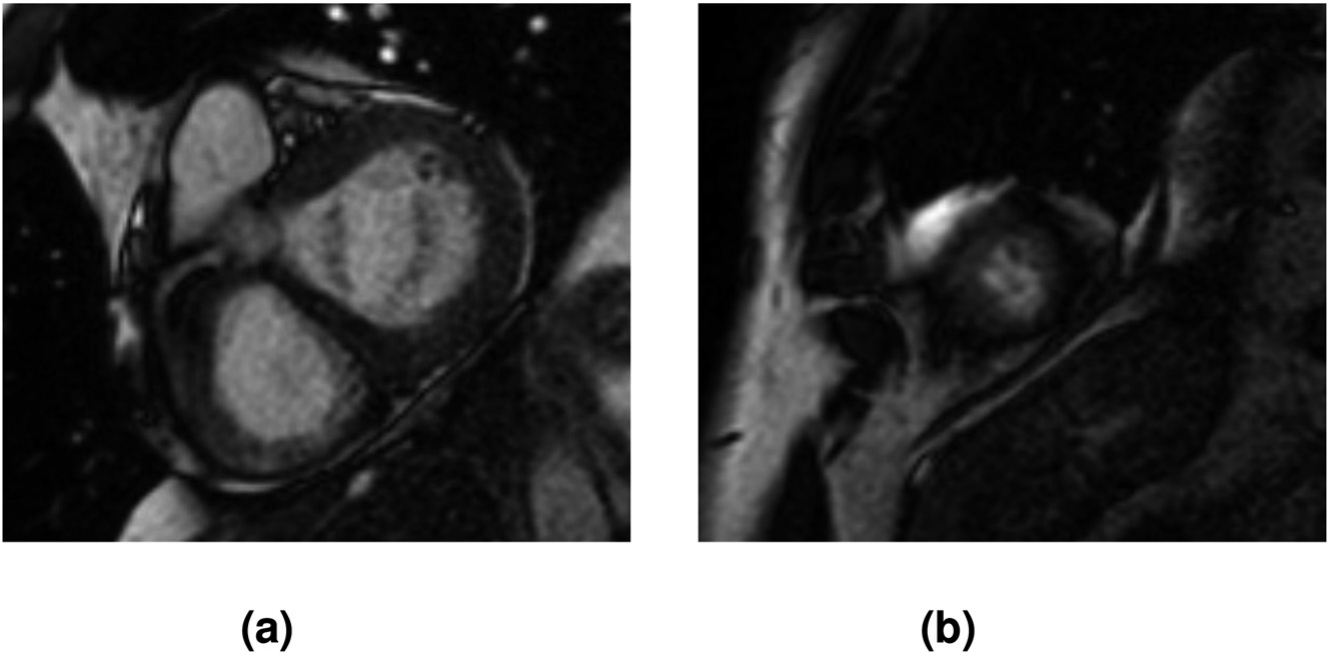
Examples of slices that cause issues for the models. (a) MRI slice no ring. (b) MRI slice showing LV with small area and artefacts.
